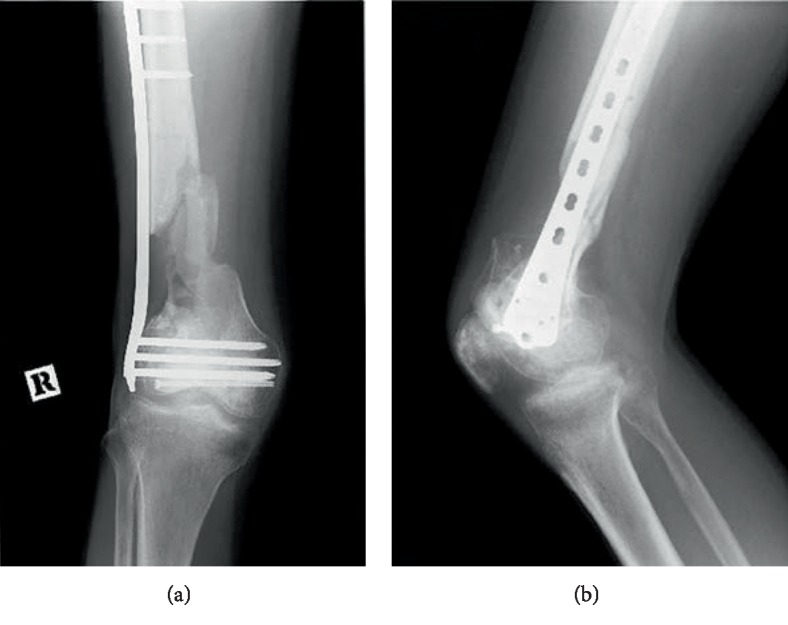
Preoperative radiograph. Anteroposterior (a) and lateral (b) radiograph of the right knee shows the metaphyseal nonunion of the femur.
